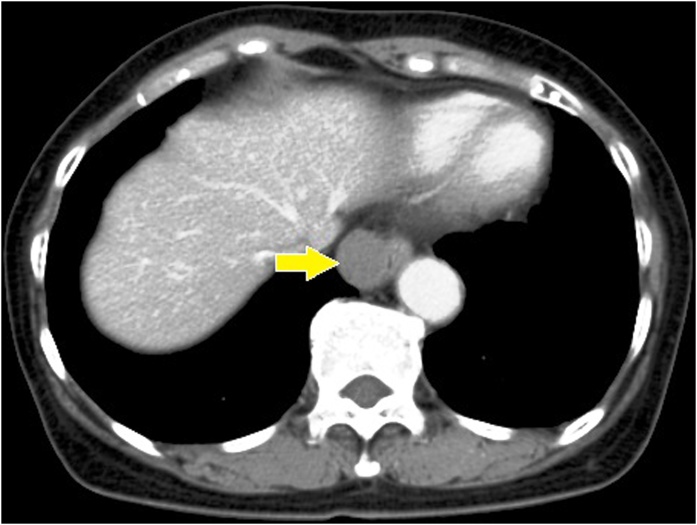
Findings from computed tomography scanning. CT showing a localized low-density area on the right side of the esophagus in the lower part of the mediastinum (arrow).
